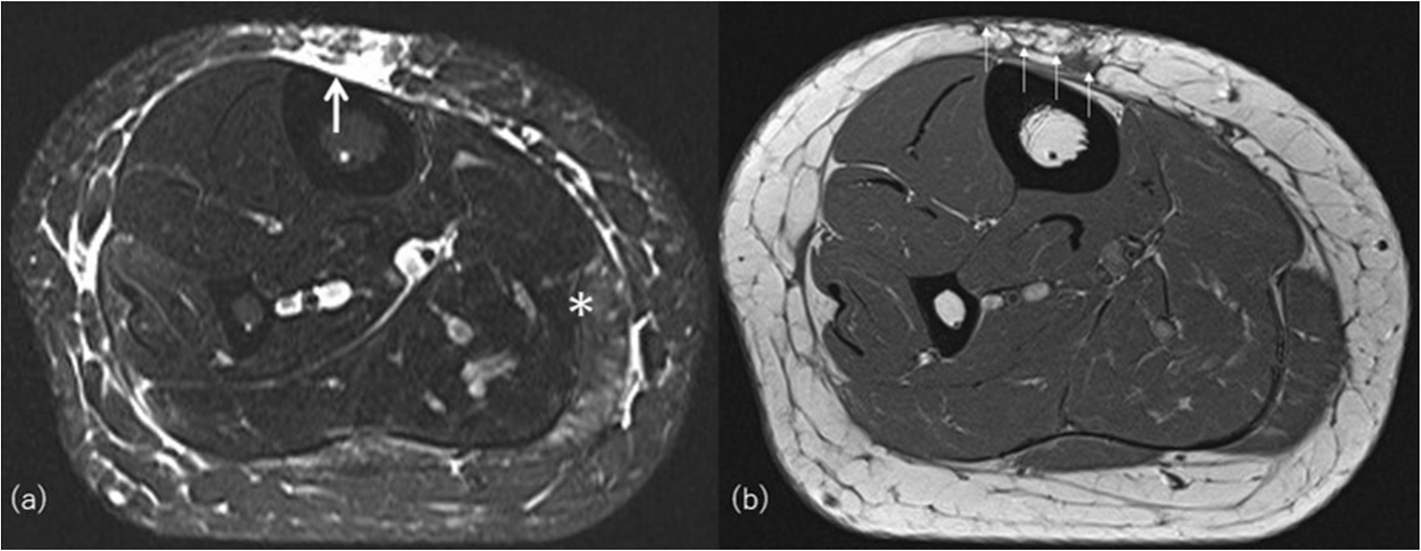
A 48-year-old woman with deep morphea on her right thigh. a Axial fat saturated T2-weighted image shows hypersignal intensity in full thickness of subcutaneous fat on the anterior aspect (arrow). Increased signal intensity in gastrocnemius muscle (asterisks), muscle fasciae, and diffuse subcutaneous septal thickening are also demonstrated. b T1-weighted image clearly shows thinning of subcutaneous fat with cord-like low signal intensity (small arrows)
